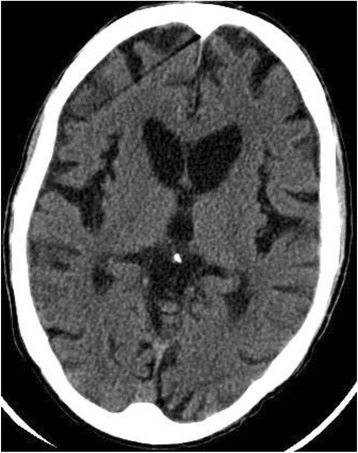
Brain CT scan of Patient III-7 showing generalized cortical atrophy and atrophy of the head of the caudate nucleus
